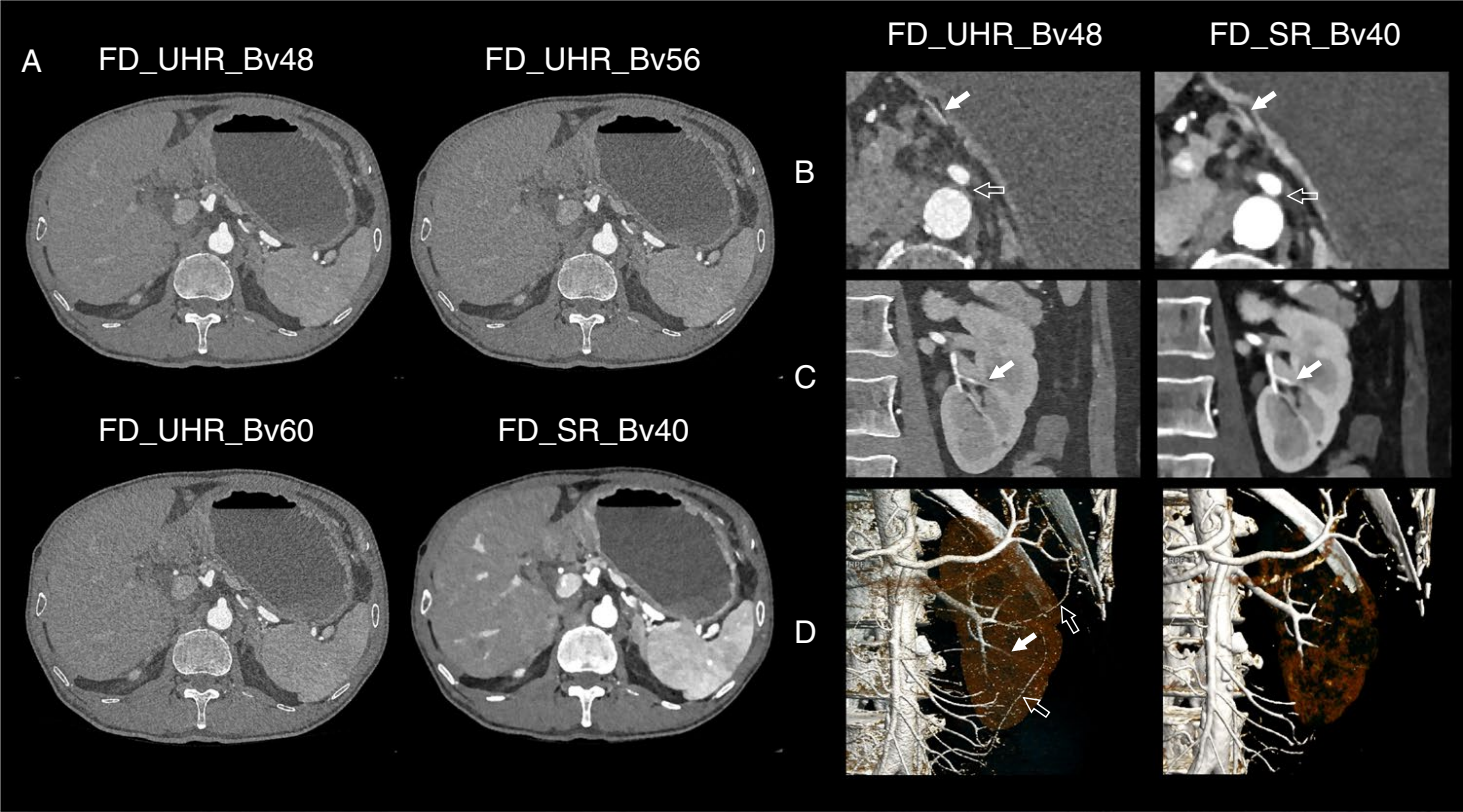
Representative example of two participants who both underwent full-dose scan. A A 71-year man with a BMI of 19.7 kg/m^2^ underwent abdominal CTA using a FD protocol with CTDIvol, DLP, SSDE, and effective dose of 6.23 mGy, 212.0 mGy·cm, 9.06 mGy, and 3.18 mSv, respectively. The data were reconstructed into FD_UHR_Bv48, FD_UHR_Bv56, FD_UHR_Bv60, FD_SR_Bv40 images, respectively. The UHR images have higher image noise, but better vessel sharpness. The overall quality of FD_UHR_Bv48, and FD_SR_Bv40 images are comparable. The FD_UHR_Bv56 and FD_UHR_Bv60 images presented better vessel sharpness than but were damaged by higher image noise. B–D Another 57-year woman with a BMI of 20.8 kg/m.^2^ underwent abdominal CTA using a FD protocol with CTDIvol, DLP, SSDE, and effective dose of 4.06 mGy, 111.0 mGy·cm, 6.25 mGy, and 1.67 mSv, respectively. The data were reconstructed into FD_UHR_Bv48, and FD_SR_Bv40 images, respectively. B The left gastric artery is only visible in axial FD_UHR_Bv48 images (solid arrow), and the soft tissue between the abdominal aorta and celiac trunk were better depicted (open arrow). C There were more visible vascular segments of left renal artery in coronal FD_UHR_Bv48 images than FD_SR_Bv40 images (solid arrow). D The left renal artery can be better visualized to the more distal vascular segments in volume-rendered FD_UHR_Bv48 images than FD_SR_Bv40 images (solid arrow). The volume-rendered FD_UHR_Bv48 images allowed more visible vascular segments of superior mesenteric artery (open arrow)
